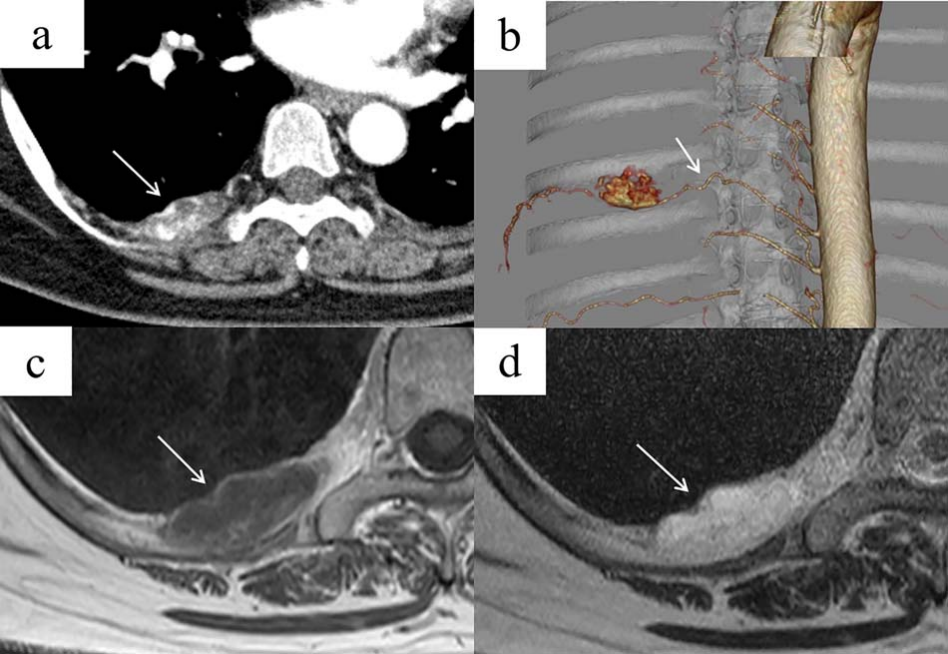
(a) Contrast-enhanced chest CT showing a right posterior mediastinal mass measuring 35 mm in diameter with heterogeneous contrast at the T7–T8 level, without extension into the spinal canal (arrow). (b) Three-dimensional reconstruction CT showing a feeding artery to the mass arising from the intercostal artery (arrow). (c, d) MRI showing low signal intensity on T1-weighted images but a bright signal on T2-weighted images (arrows). A high-signal area on T1-weighted MRI is suggestive of a fatty component inside the tumor. (c) T1-weighted image. (d) T2-weighted image.
